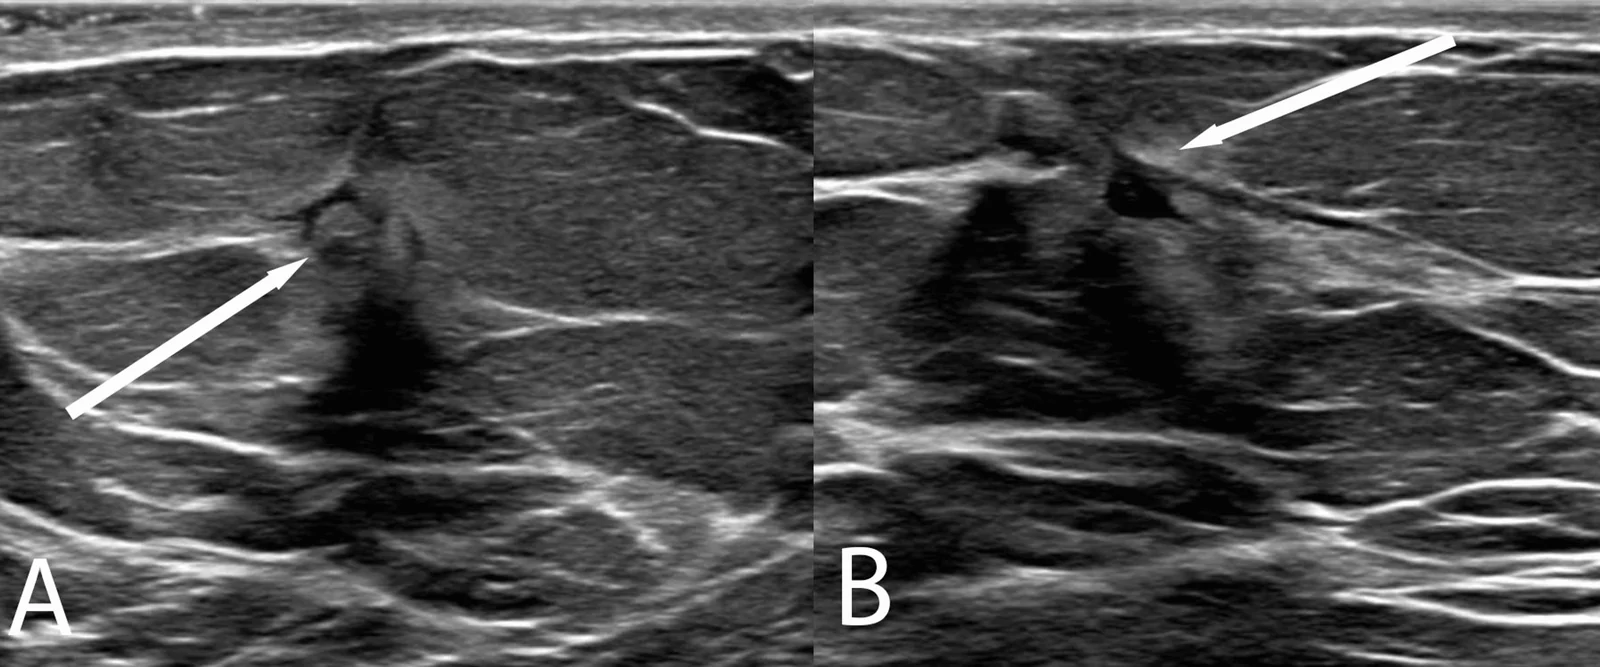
Targeted ultrasound of the right breast performed on the same day as the diagnostic mammogram. (A) Longitudinal and (B) transverse images of the right breast at 4:00 demonstrate an irregularly shaped, mixed-echogenicity mass with angular margins measuring 1.8 cm (white arrows). This was classified as suspicious and given a Breast Imaging Reporting and Data System (BI-RADS) assessment category 4 on ultrasound. Ultrasound-guided biopsy was subsequently recommended.
